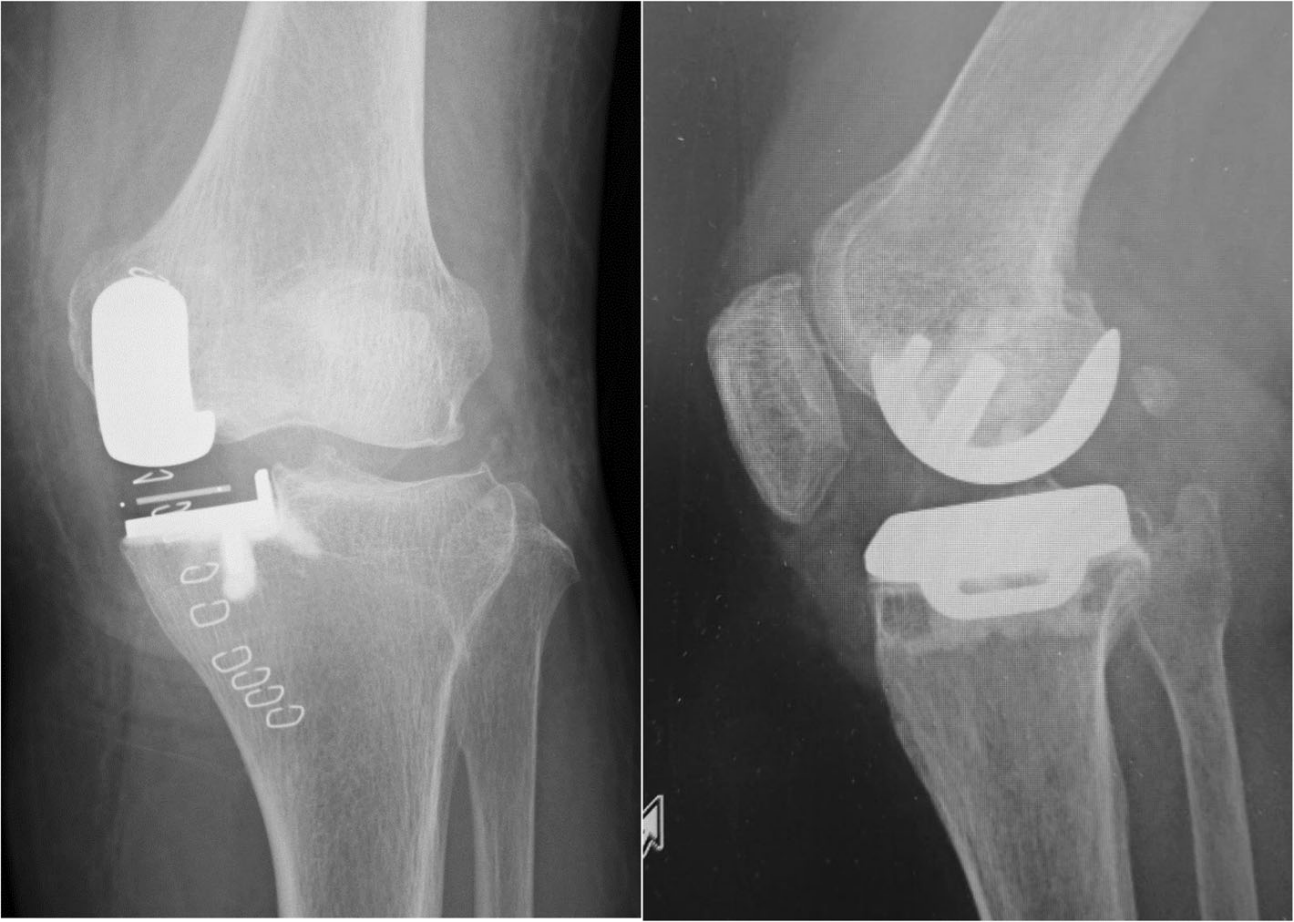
Radiographs of case 1 after the revision surgery
